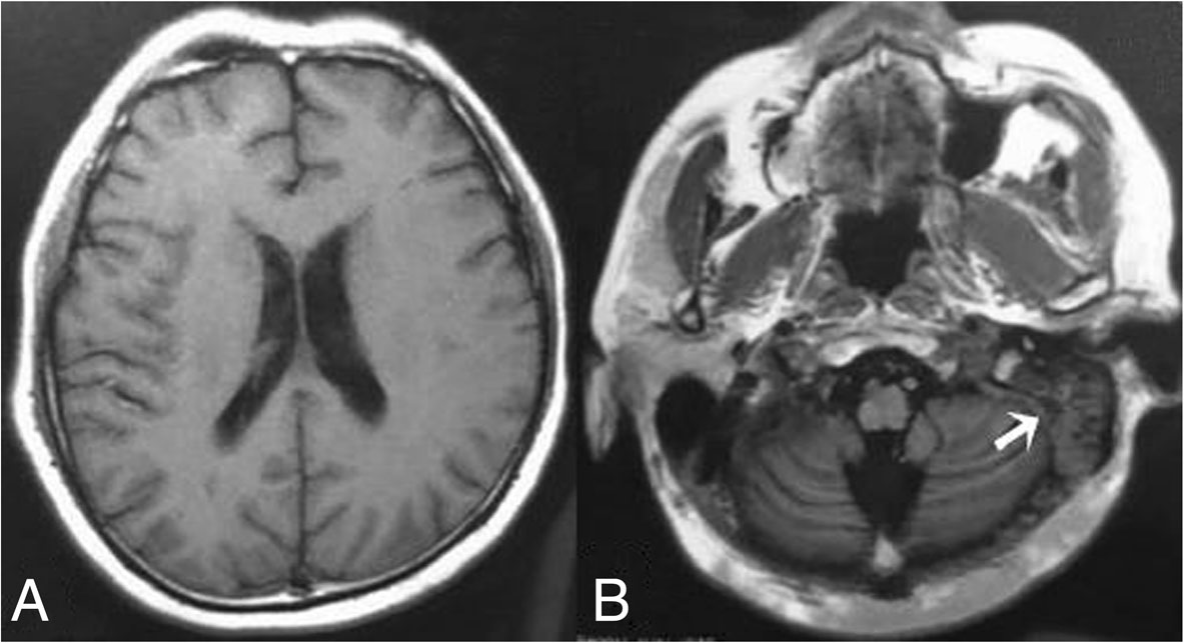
MRI at the junior hospital showed turbid sulcus (a) and OM in the left ear (arrows in b)
